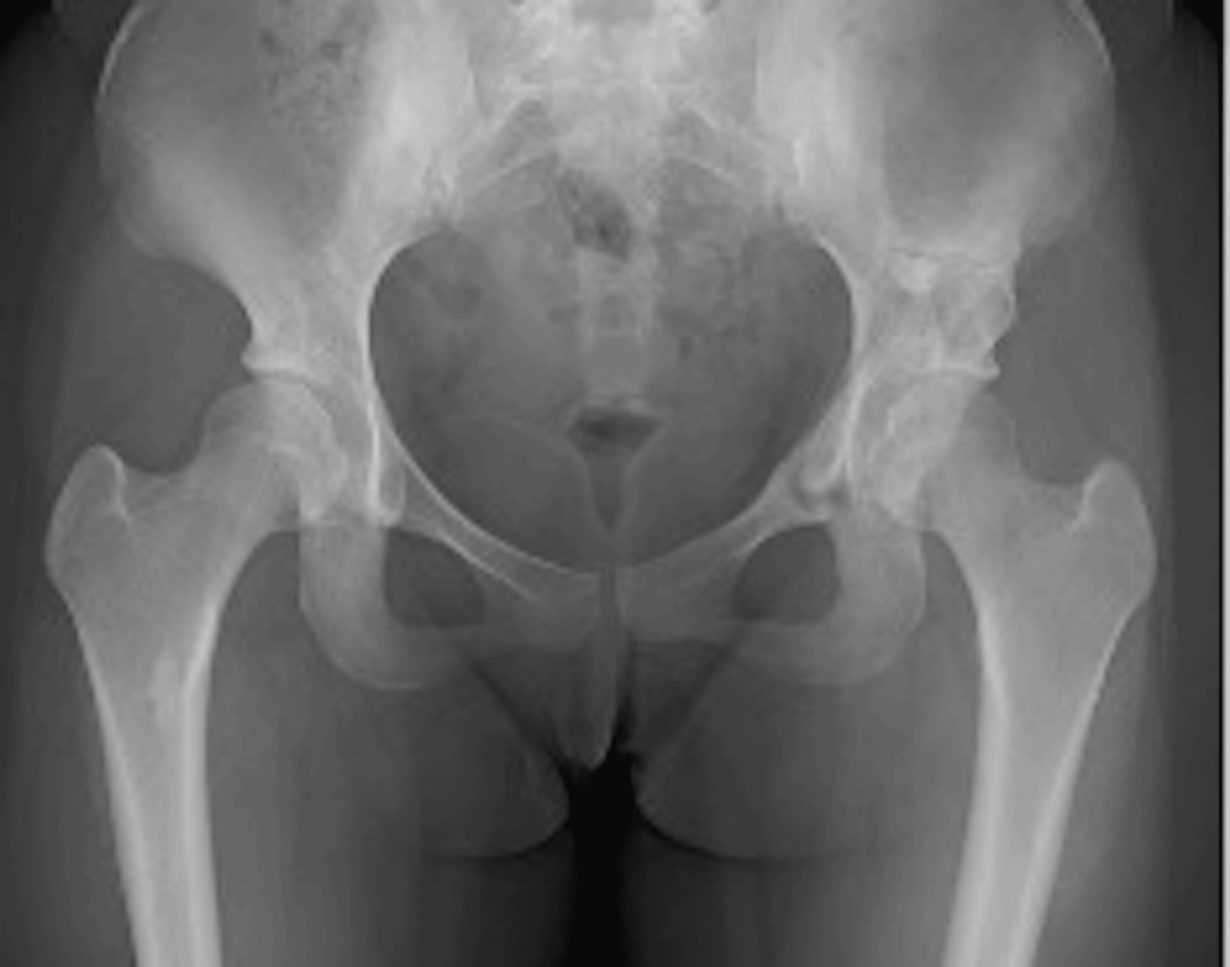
Radiographs at six months postoperatively of case 2. Complete bone union was observed at the osteotomy site. CE angle: 25.0°, ARO: -0.9°, Sharp's angle: 51.1°, AHI: 87%. CE, center–edge; ARO, acetabular roof obliquity; AHI, acetabular head index
